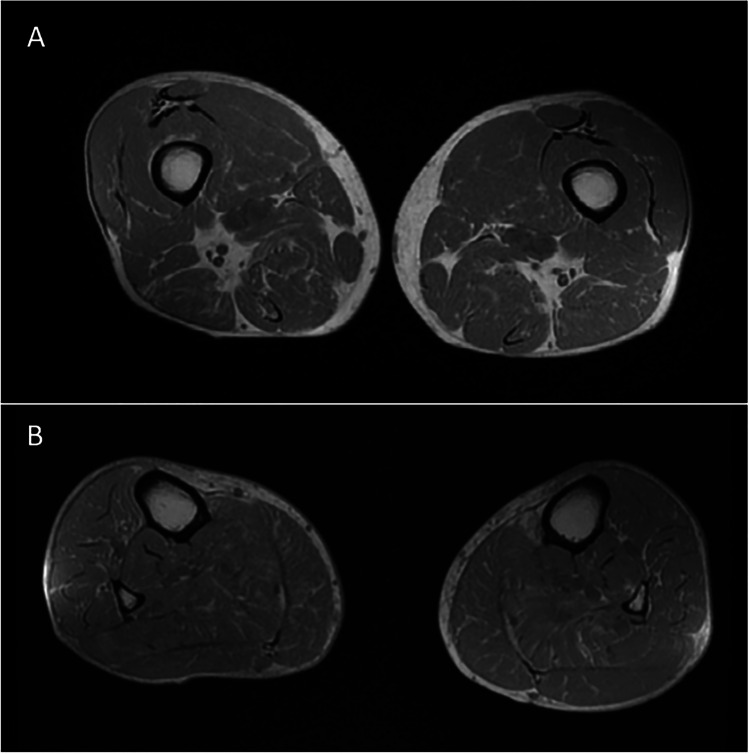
Ax IN/OUT MRI sequences of our case showing signs of fatty infiltration in the posterior compartment of the thighs (A) and in the anterior and posterior compartment of lower legs (B)
